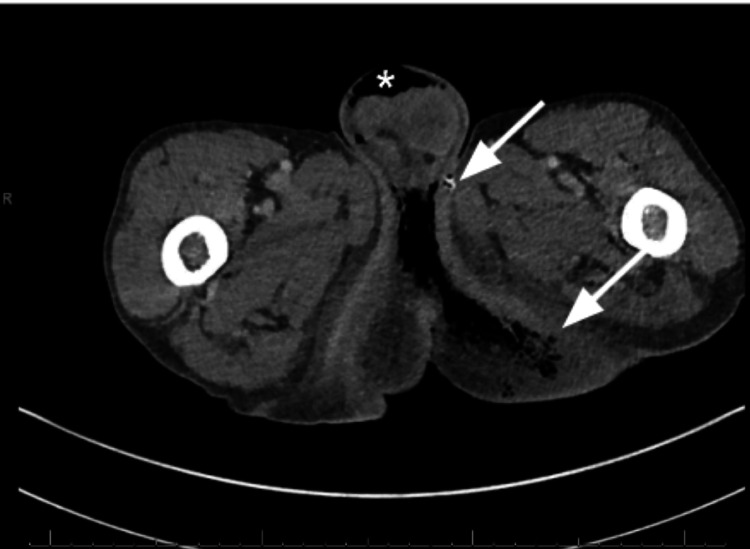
Axial enhanced CT image shows soft tissue edema and fascial thickening (*) in the bilateral scrotum and perineum extending into the left buttock (arrows).
